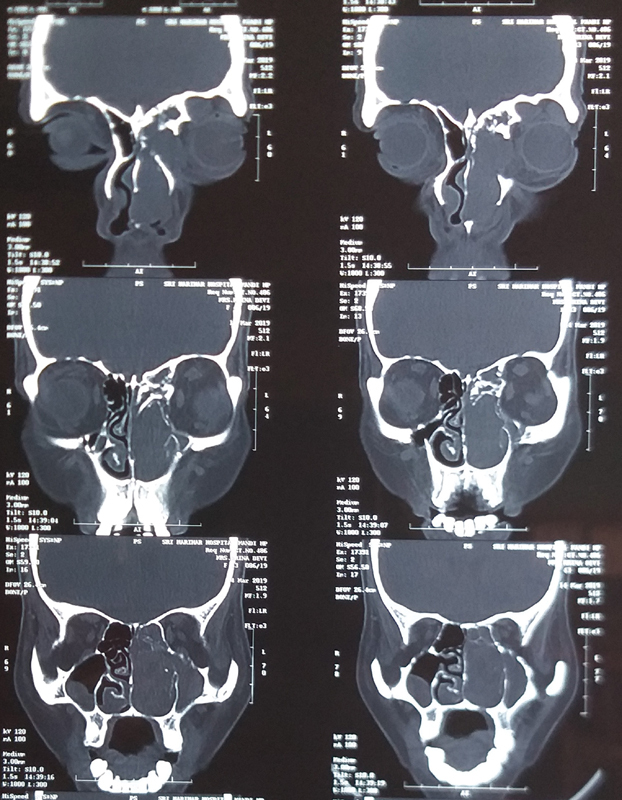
Computed tomography scan showing isodense expansile mass in left nasal cavity, ethmoids, frontal sinus, and orbit.
